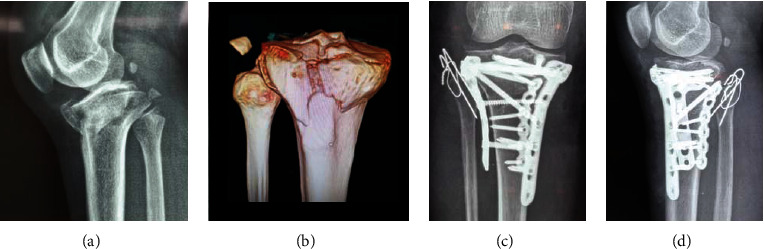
A type 1 case fixed with Kirschner wire by tension band technique: (a) preoperative lateral X-ray; (b) preoperative CT; (c, d) preoperative X-ray.
